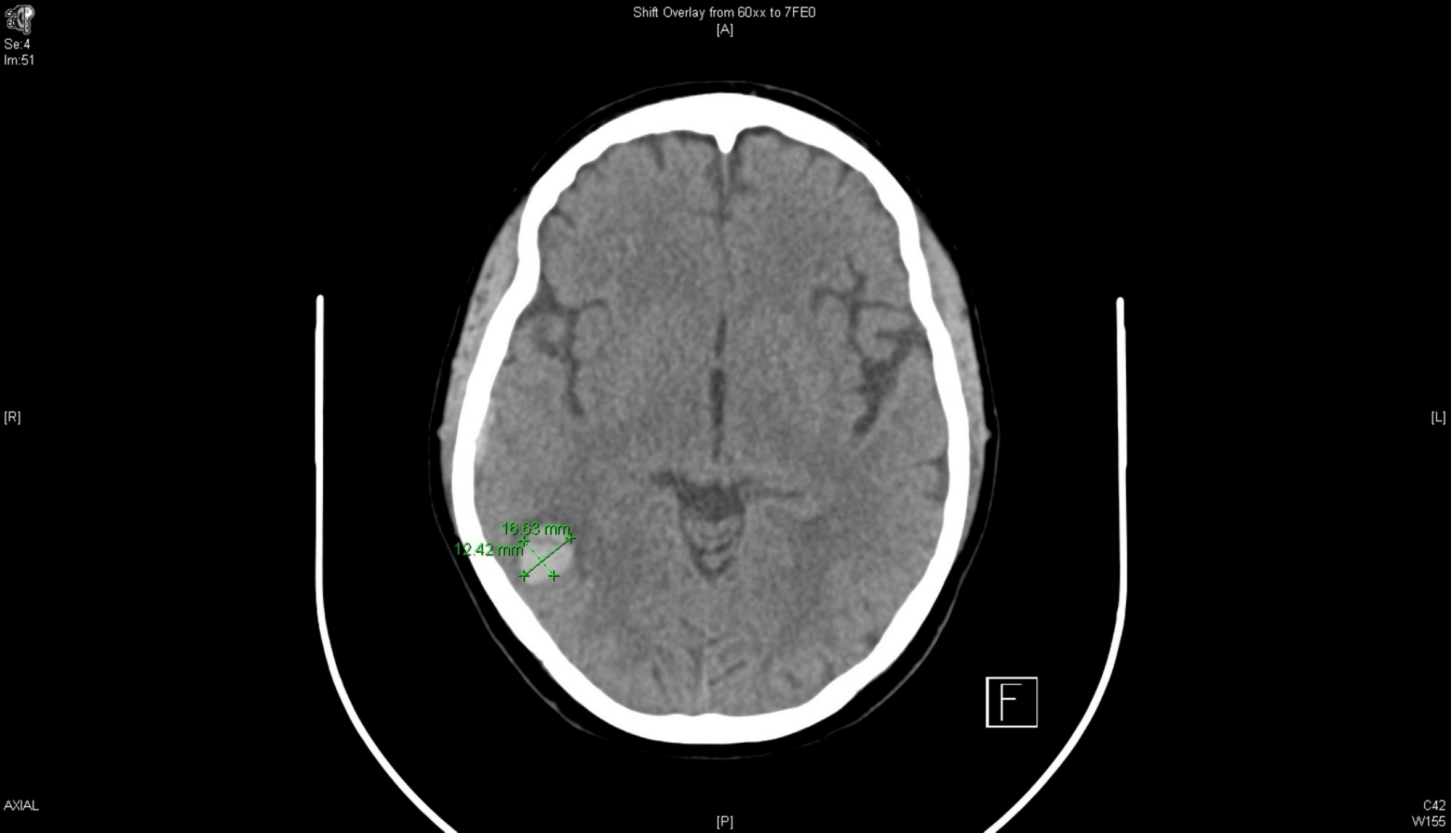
Computed tomography (CT) head without contrast, day 3.
